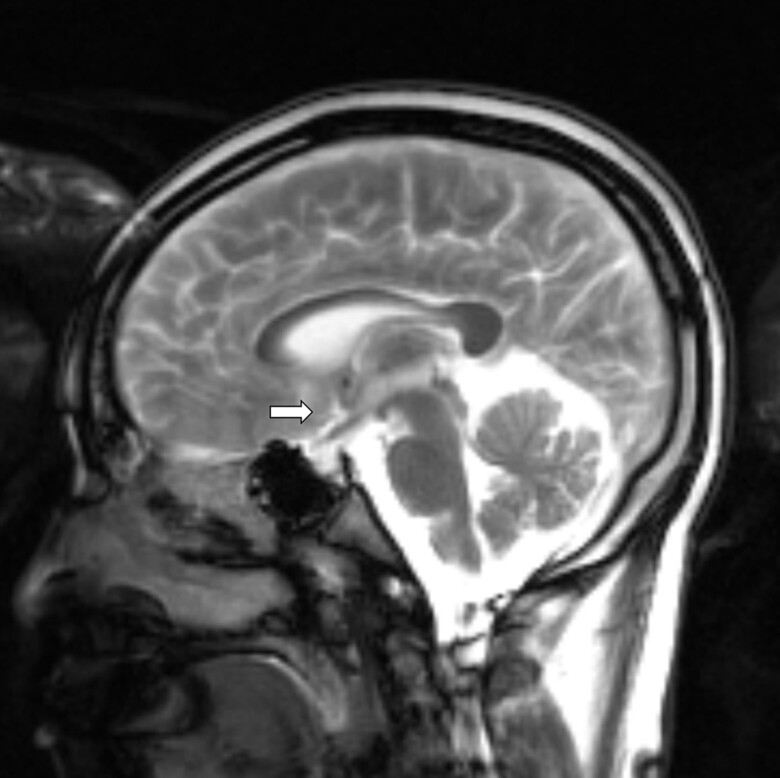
Brain magnetic resonance imaging with numerous small nodular enhancement involving leptomeninges and cerebrospinal fluid space supratentorially and infratentorially, pituitary stalk (arrow), interpeduncular cistern, and periaqueductal perivascular space.
